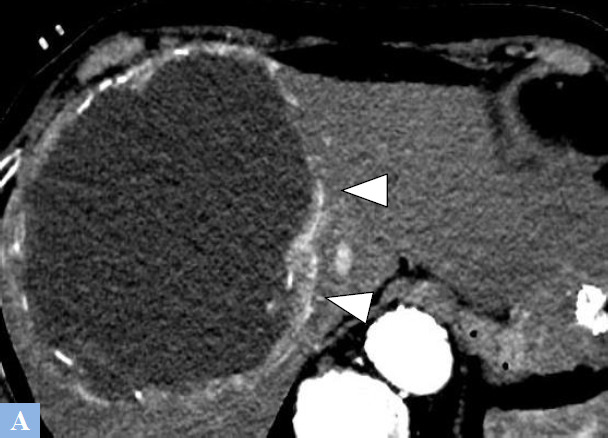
Cystic metastasis from a gastric GIST. CT image in the axial plane at portal phase of enhancement showing mixed lesion, both solid and cystic, in the right liver with a hypervascular wall (arrowheads).
